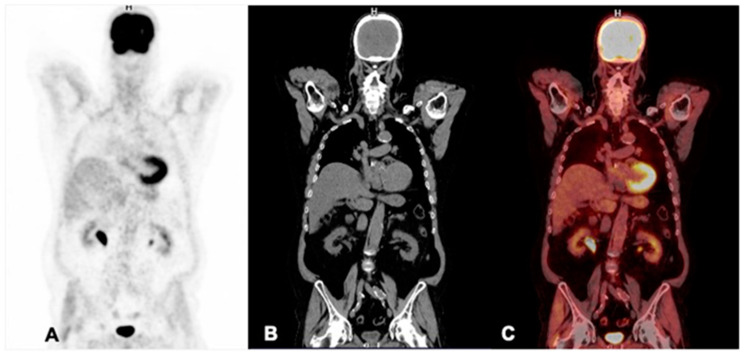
Example of PET/CT images using the radiopharmaceutical ^18^F-FDG of a prostate cancer patient. The PET/CT images allow the simultaneous evaluation of biochemical and biological processes of the whole body (through the PET component) and anatomy (through the CT component). The metabolic images of PET are fused with the anatomic images of the CT scan. The coronal images of the (A) PET, (B) CT, and fused (C) PET/CT images demonstrate normal uptake and no metastases in this study.
